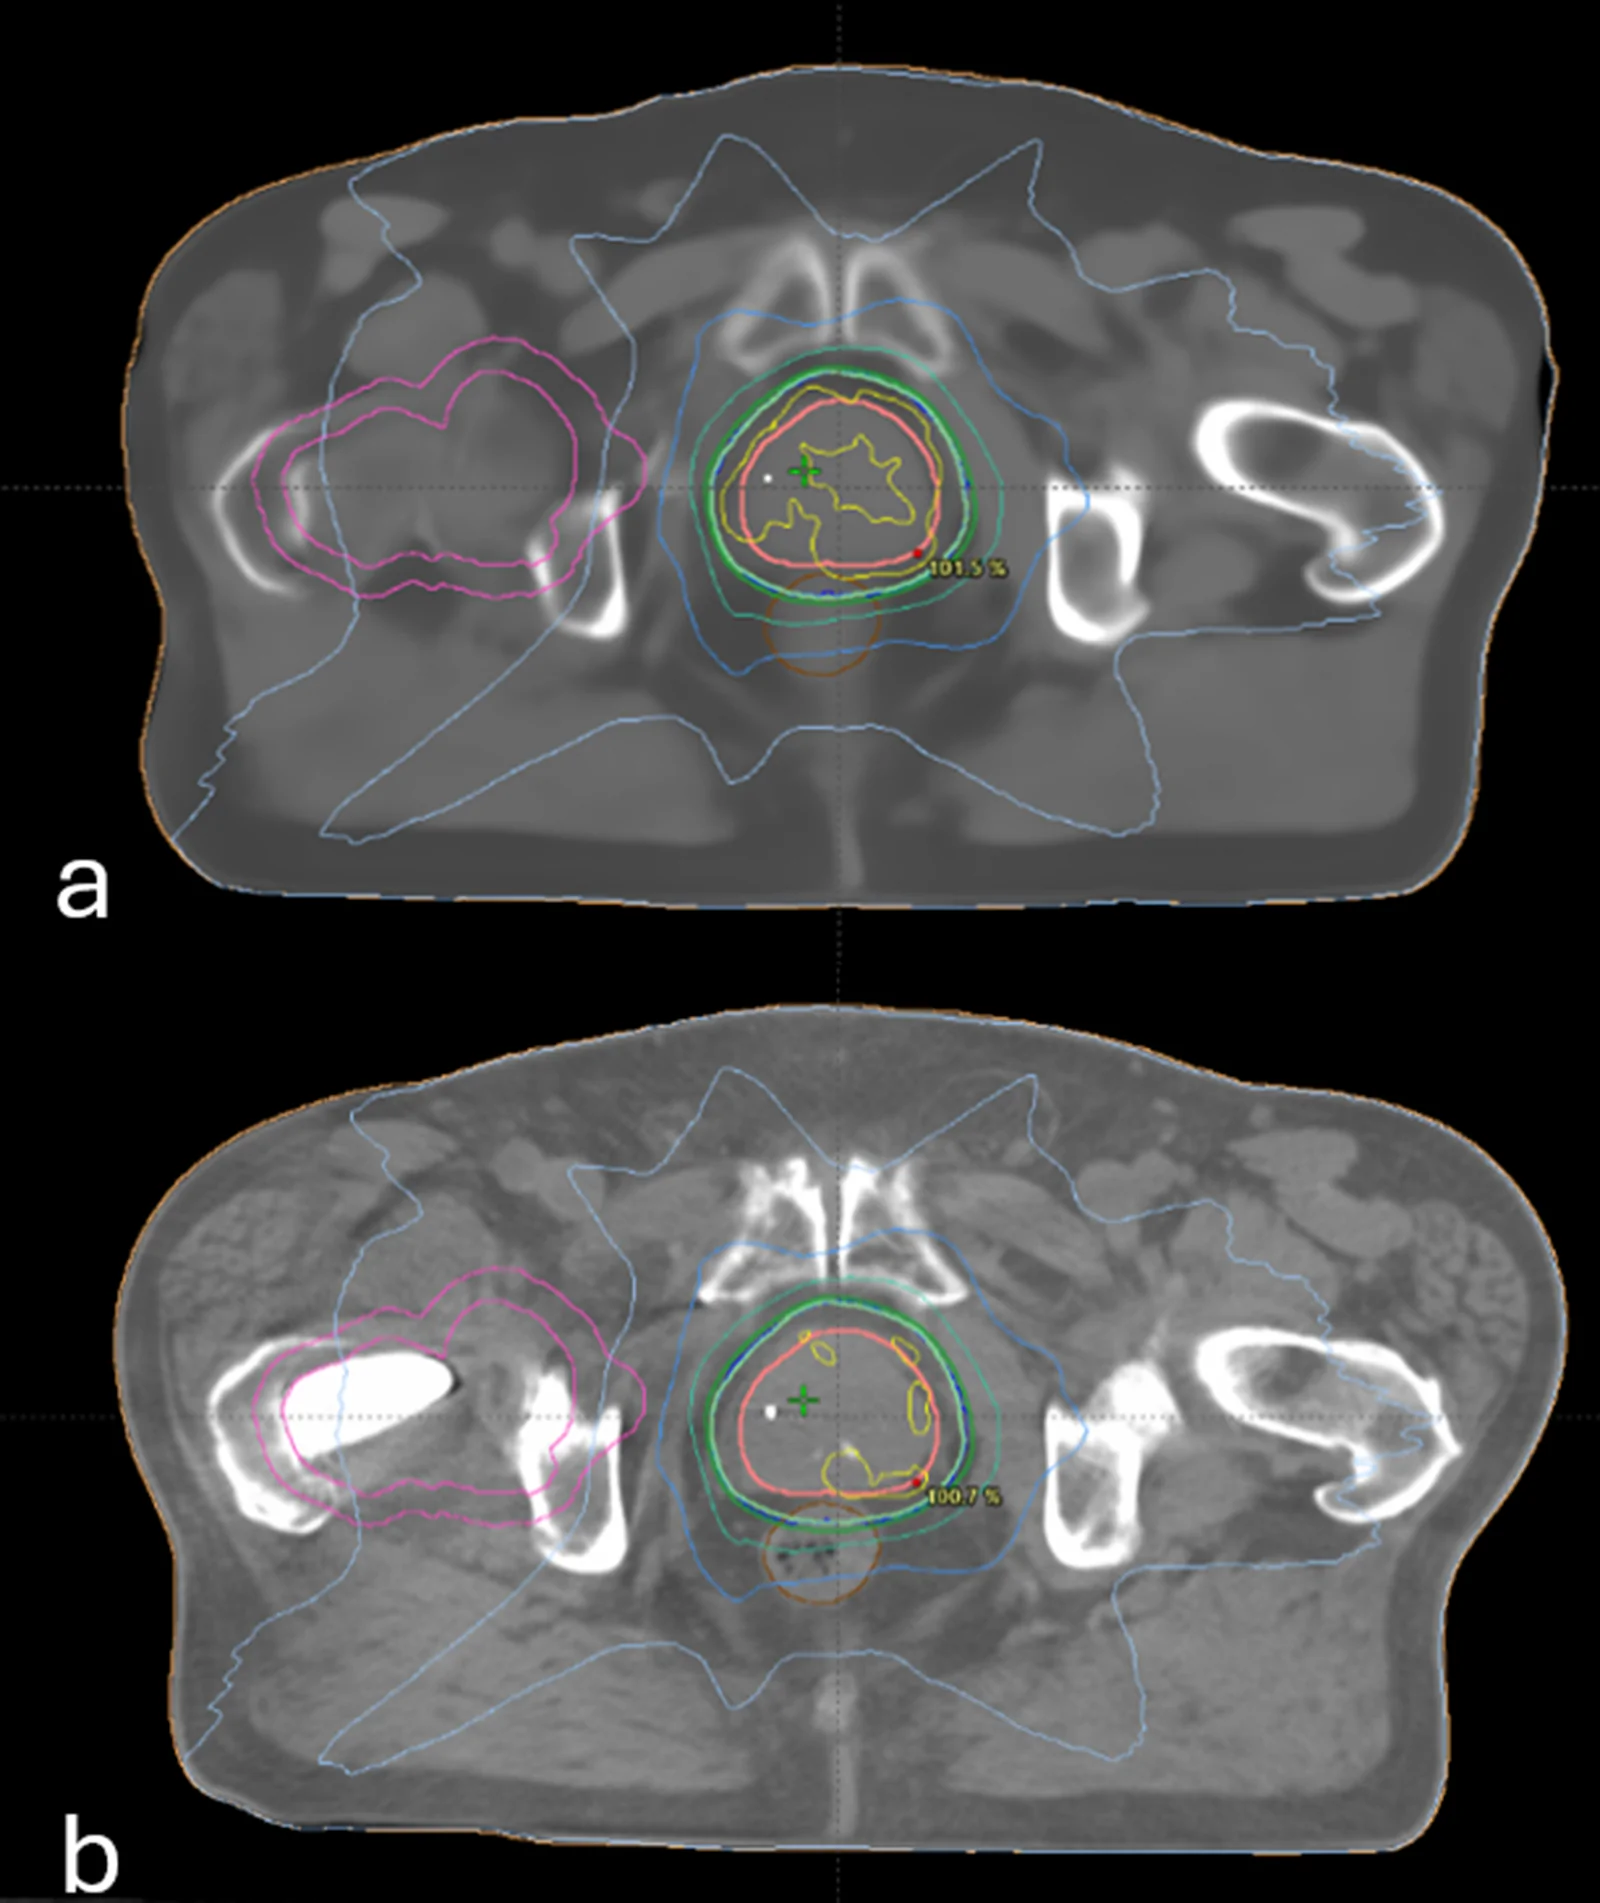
(a) Treatment plan optimized on sCT using an MR-derived avoidance sector. (b) Corresponding plan recalculated on CT after rigid registration in Hero, using the initial registration from Eclipse
